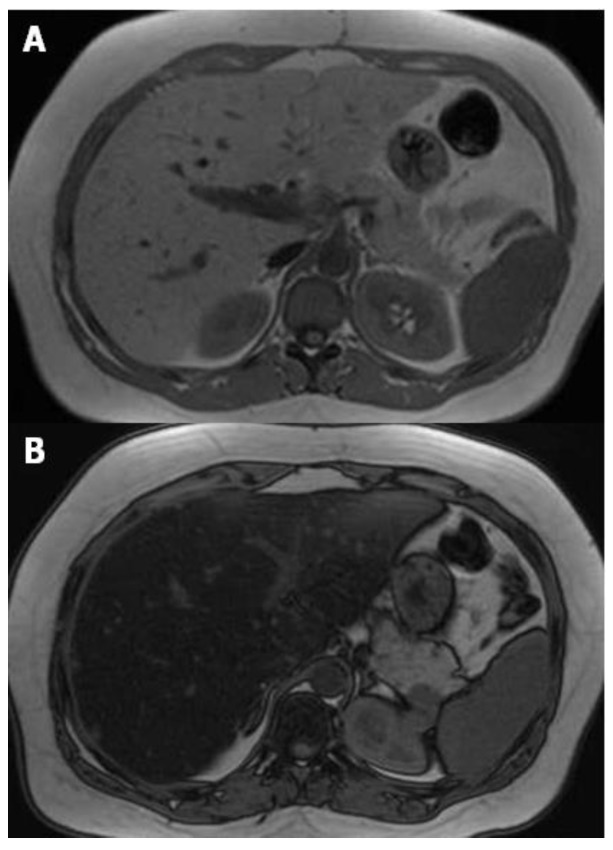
MR T1-weighted images with different echo times (TE) 4.2 (A) and 2.1 (B), show a marked drop of signal intensity in the out-of-phase image (B) which is a sign of severe steatosis.
